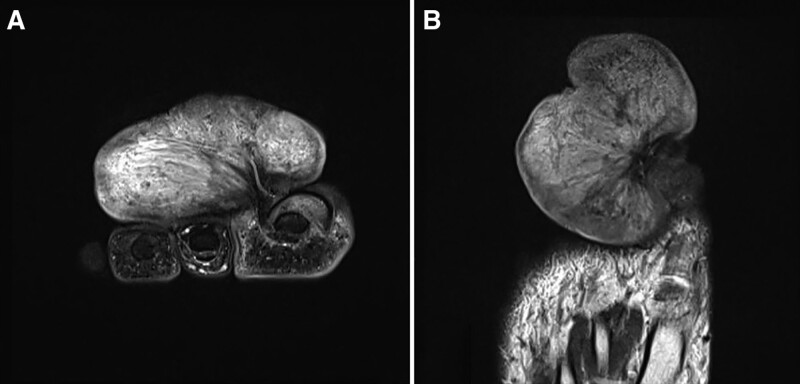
(A) Coronal and (B) axial images of MRI T2WI of the right toe, showing a huge fibrous component soft tissue mass originating from the lateral side of nail bed.
